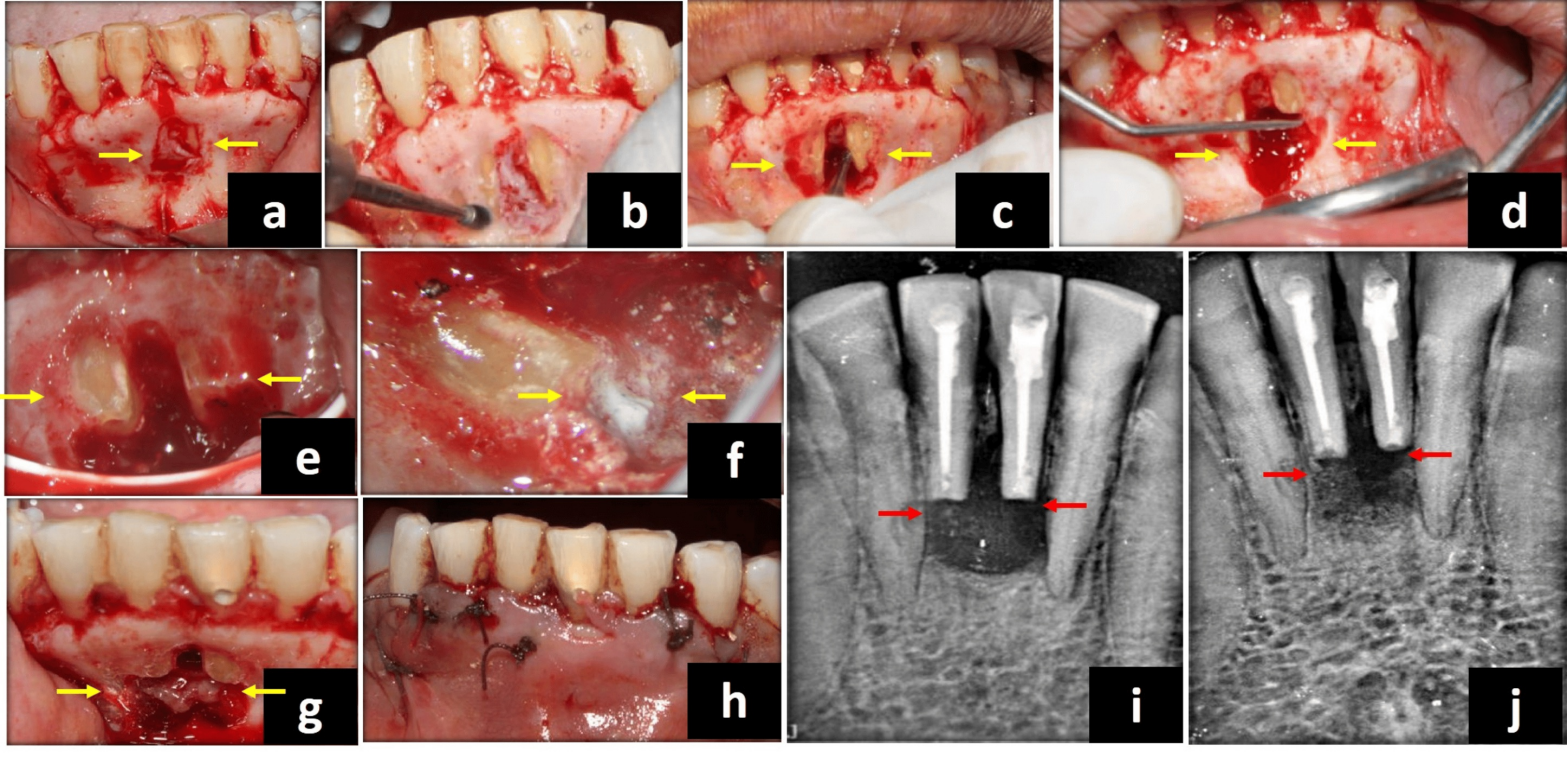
Surgical procedure (a) Full-thickness mucoperiosteal flap elevated between mesial line angle of teeth 33 and 43. (b) Bony window prepared using round carbide bur. (c) Root-end resection of the apical third with a thin straight #701 fissure bur. (d) Use of ultrasonic retro-tips for retro-cavity preparation. (e) Retro-cavity prepared. (f) MTA retro-fill. (g) CGF placement over the lingual perforation site into the bony cavity. (h) Multiple interrupted sutures placed to approximate the flaps. (i) Intra-operative IOPA radiograph to confirm the MTA condensation into canals and no over-fill. (j) Immediate post-surgical IOPA radiograph. MTA: mineral trioxide aggregate; CGF: concentrated growth factor; IOPA: intra-oral periapical
